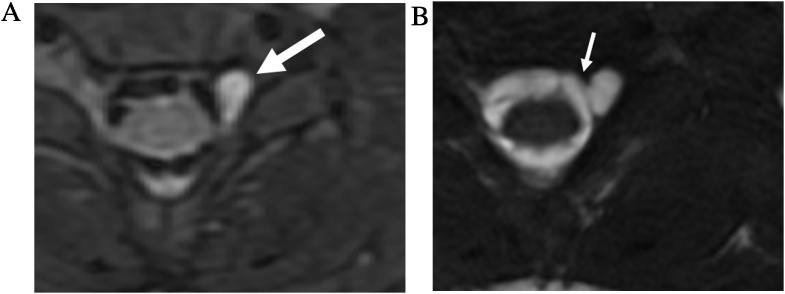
Brachial plexus birth injury. 6-month-old male with pseudomeningocele. Axial T2-weigted fat saturated FSE MRI image (a) demonstrates CSF intensity cyst in the left C6-7 neuroforamen. Intradural nerve roots are obscured by pulsation artifact. Axial FIESTA-C MRI image (b) shows the site of dural defect (thin arrow) and associated pseudomeningocele. The left sided intradural rootlets and exiting left C7 nerve root are absent, indicating avulsion.
